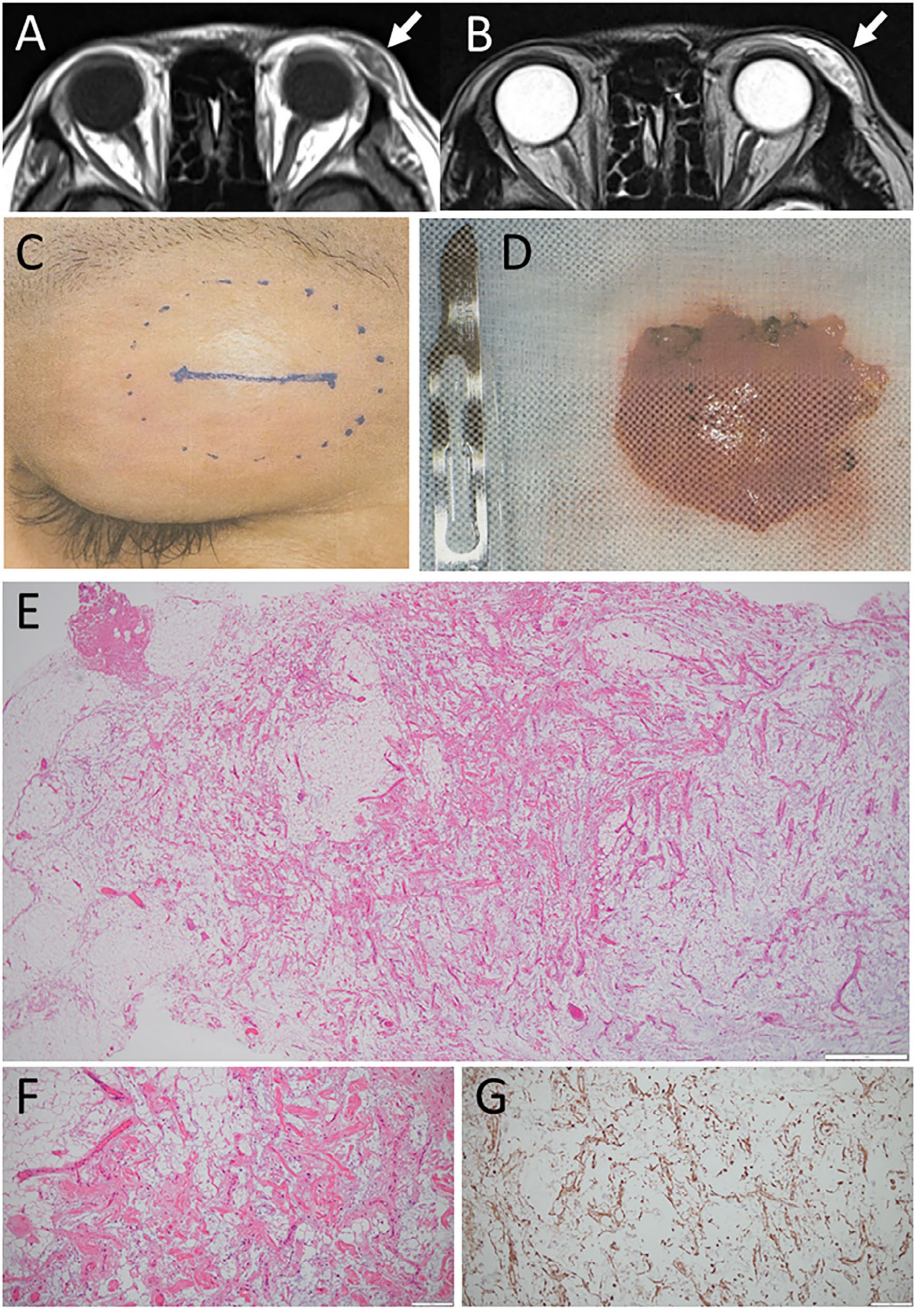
Left upper eyelid saucer‐shaped mass with inhomogeneous content on T1‐weighted axial image (arrow, A) and T2‐weighted axial image (arrow, B) of magnetic resonance imaging. Left upper eyelid subcutaneous flat mass with 2 × 2.5 cm in size at surgery (C) and macroscopic view of the extirpated mass with size reference of no. 15 curved blade (D). Note incision line and area of the mass are marked on the skin at surgery by local anesthesia (C). Collagen fibers, capillaries and small vessels, and spindle cells in small number which are dispersed among mature adipose tissues with varying sizes (E in low magnification, F in high magnification). Spindle cells are positive for CD34, supporting the diagnosis of spindle cell lipoma. Bar = 1000 μm in E, 200 μm in (F and G).
